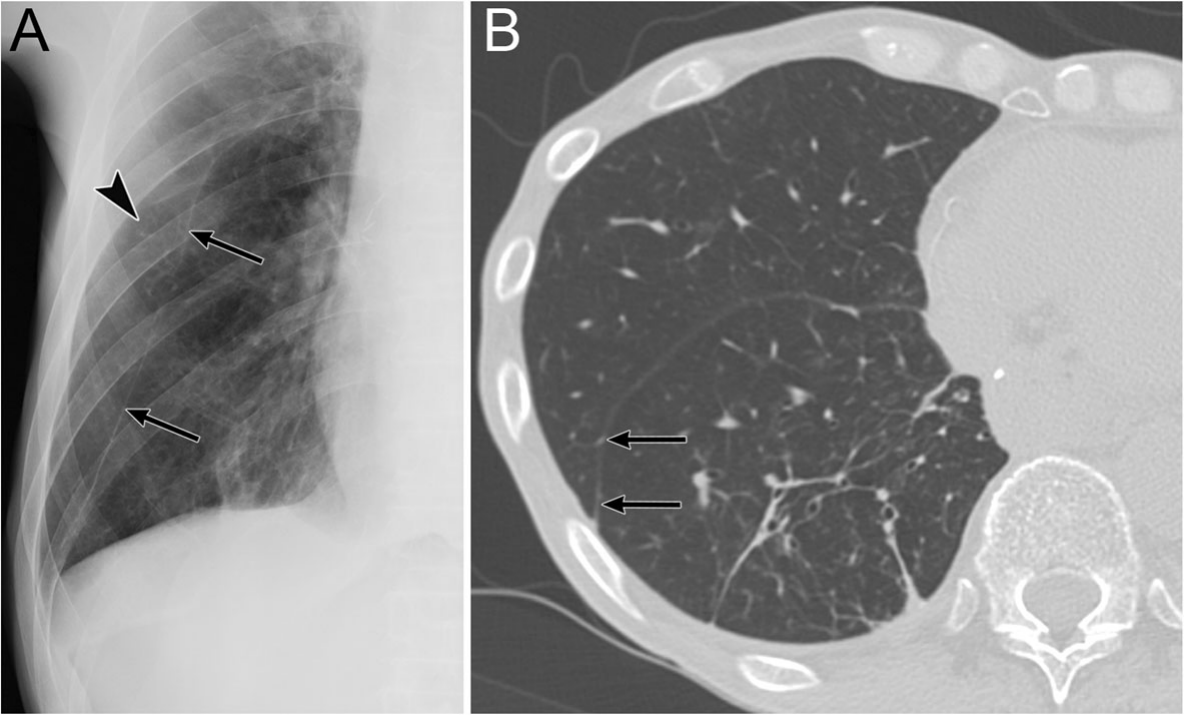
The major fissure mimicking pneumothorax on a radiograph in a 71-year-old man. He underwent partial resection of the right S6 for lung metastasis of esophageal cancer. a The major fissure appears as a thick linear opacity (black arrows) in the right lung field on a supine radiograph. It resembles the visceral pleura in pneumothorax. Unlike pneumothorax, vascular markings (black arrowhead) are visible outside of the thickened fissure. b An axial CT image shows the major fissure extending in the anteroposterior direction with a slight oblique angle (black arrows). Some volume loss in the right lower lobe caused the fissure to be displaced posteriorly; therefore, it seems to be visible on the anteroposterior radiograph
